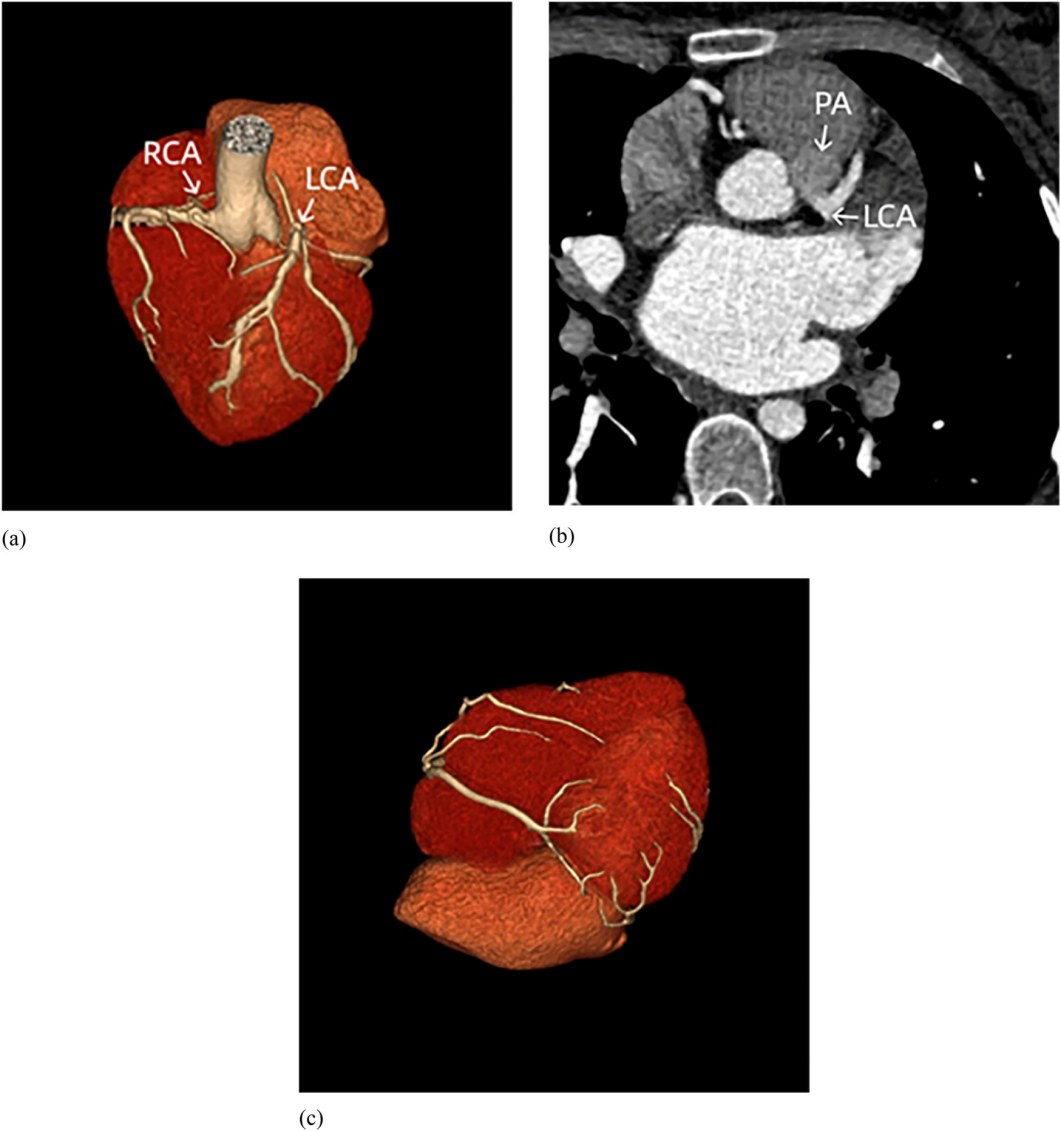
Coronary CT. (a) The right coronary artery (RCA) originates from the aortic root, while the left coronary artery (LCA) originates from the pulmonary artery (without pulmonary artery reconstruction). (b) The LCA originates from the pulmonary artery (PA). (c) Extensive communications exist between LCA and RCA.
